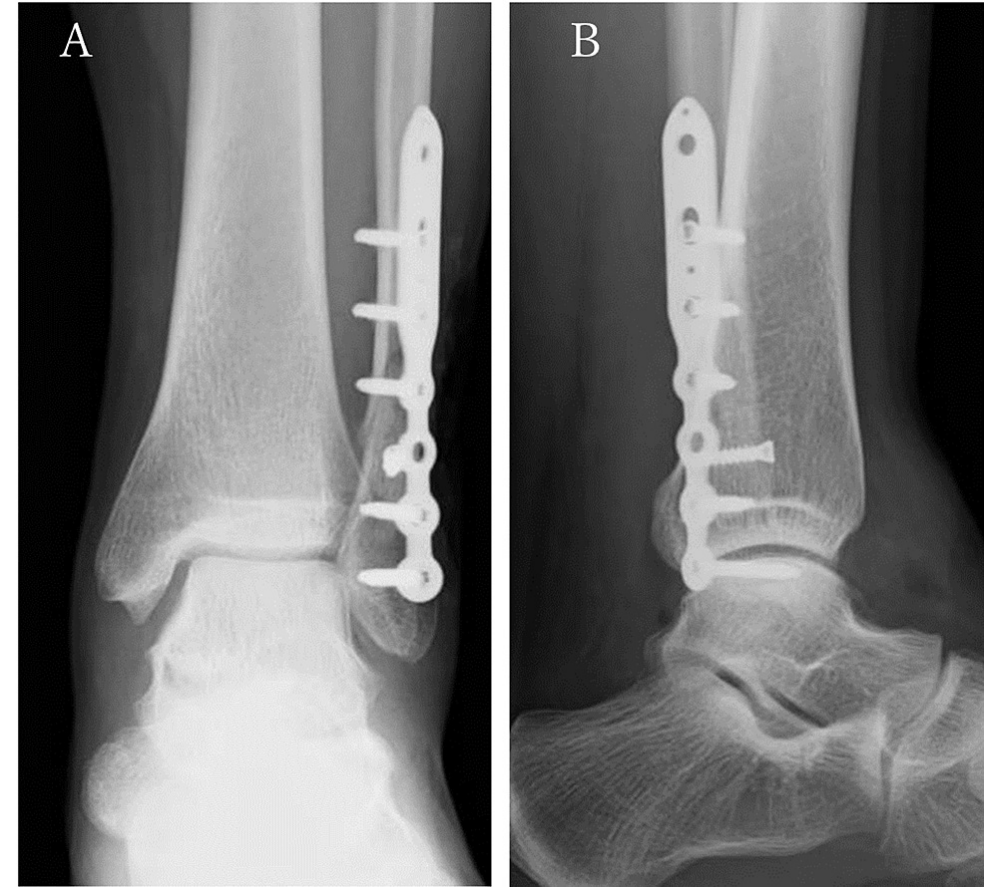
The patient's postoperative radiographs (A) Frontal view; (B) Lateral view
